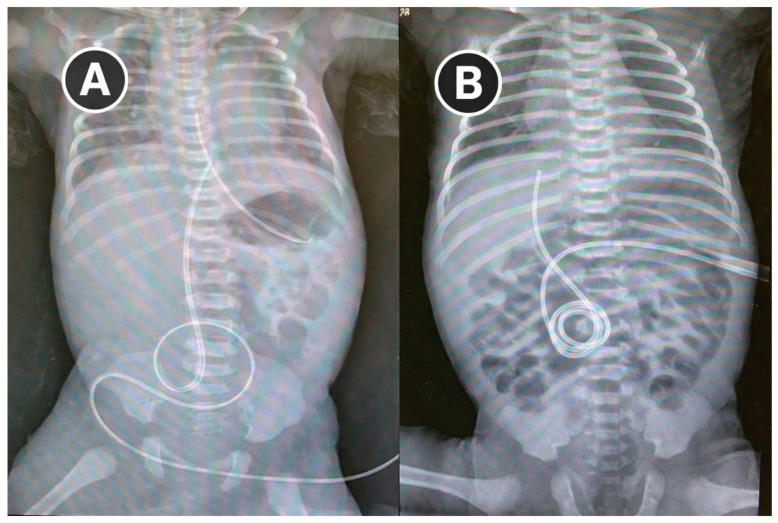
Progressive increase in bowel gas within the intestinal loops in a neonate with uncomplicated meconium ileus. (A) Radiograph obtained a few hours after birth, after placement of a nasogastric tube and an umbilical catheter; (B) Radiograph obtained 3–4 days later, demonstrating progressive intestinal gas filling.
